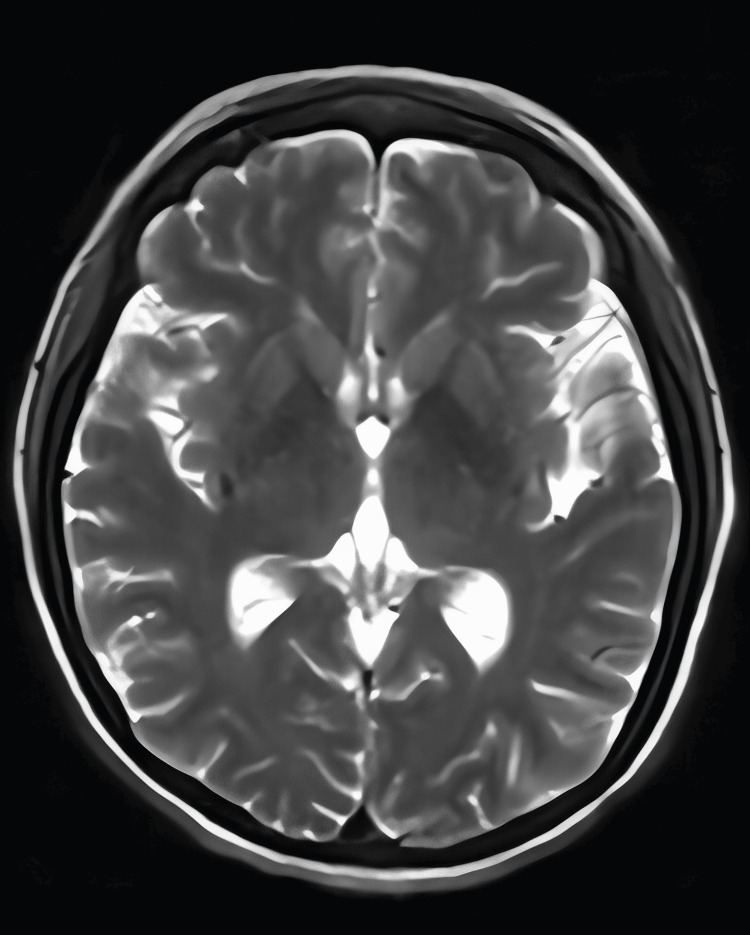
Brain MRI Imaging revealed heterogeneous diploic marrow with a discrete diffuse hyperintense area toward the bilateral frontoparietal convexity on T2 and FLAIR sequences, associated with cortical hyperintensity and diffusion-related T2 shine-through in the left frontoparietal, right frontal, and left temporo-occipital regions, alongside leptomeningeal enhancement of the left cerebral hemisphere and right temporo-occipital region, and continuous dural enhancement with supratentorial thickening.
